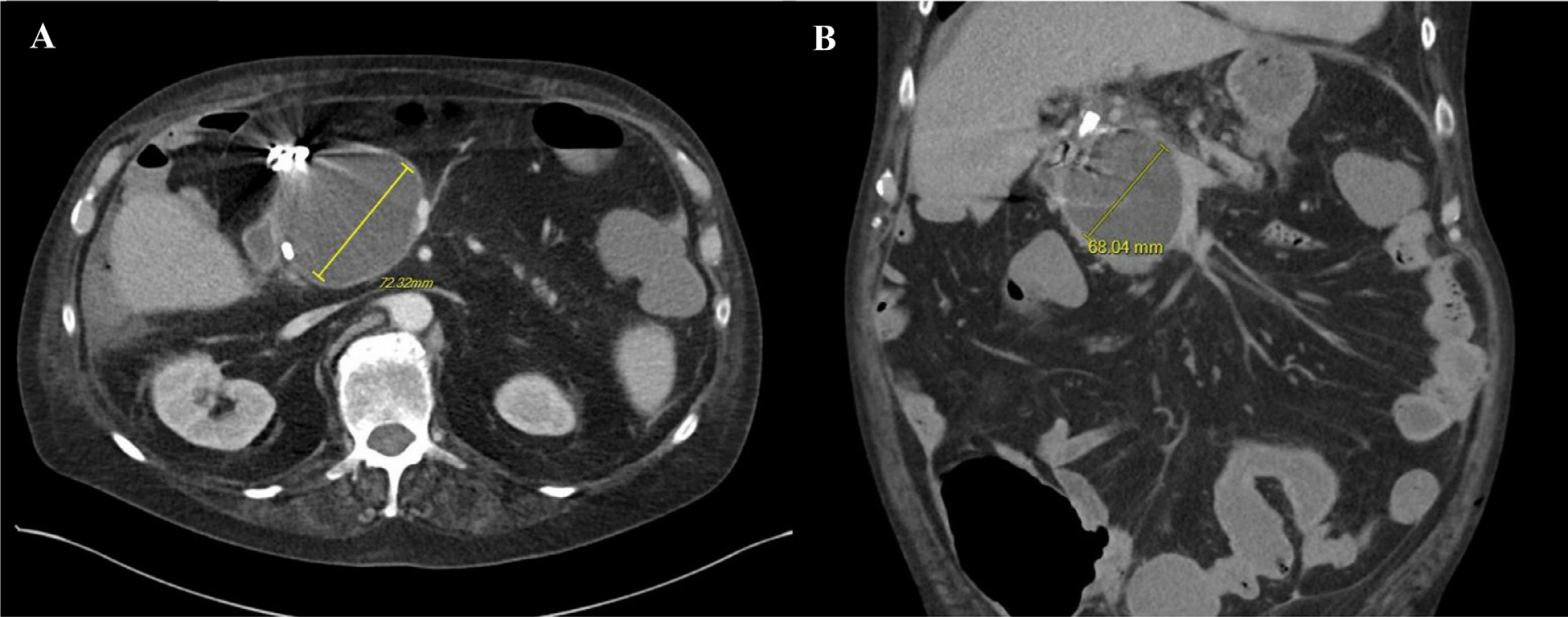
(A and B) Postoperative pseudoaneurysm visualized on axial and coronal CT images. A solid hypodense area corresponds to the neck of the gastroduodenal artery pseudoaneurysm, which has been successfully occluded with embolization packing and Ruby coils. No residual arterioportal fistula is evident. The pseudoaneurysm measured 72.32 mm in the axial plane and 68.04 mm in the coronal plane, indicating regression from embolization interventions performed.
